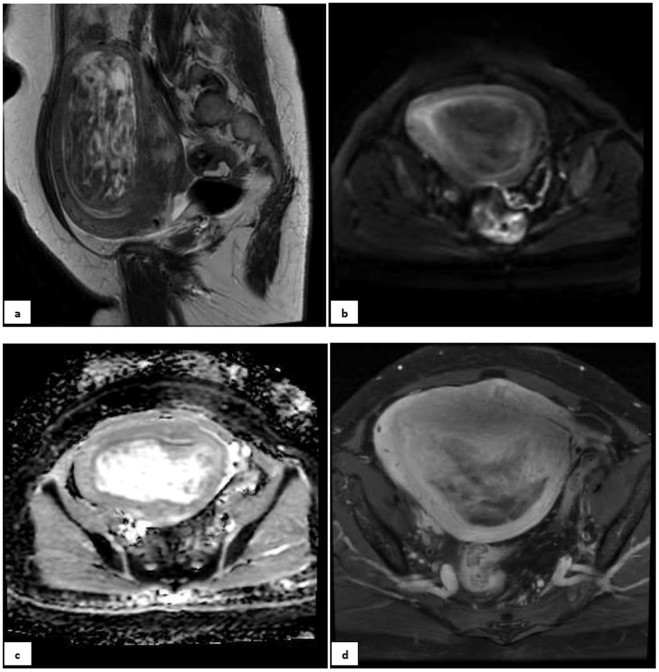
A 32-year-old nulliparous patient with 6 months of abnormal uterine bleeding and a heterogeneous myometrial mass on her ultrasound. (a) Sagital T2 MR-image detected a large predominantly hyper-signal lesion in posterofundal myometrium with anterior endometrial displacement; Tumor-Myometrial Contrast (TM) Ratio = 0.83; T2-scaled Ratio = 0.2 and T2 map = 42. (b) Axial DW image and (c) ADC revealed no evidence of restriction with mean ADC of 1.3 mm/s^2^. (d) Axial post-contrast T1 image in equilibrium phase showed the mass with mild enhancement significantly less than myometrium and without any central necrosis. Tumor-Myometrium Contrast Enhanced (TMCE) Ratio = −0.64. If we put this sample data in either of simple or complex decision-tree, the pathology could be predicted as “benign”. Eventually, the histo-pathological exam confirmed a benign tumor, degenerated leiomyoma.
